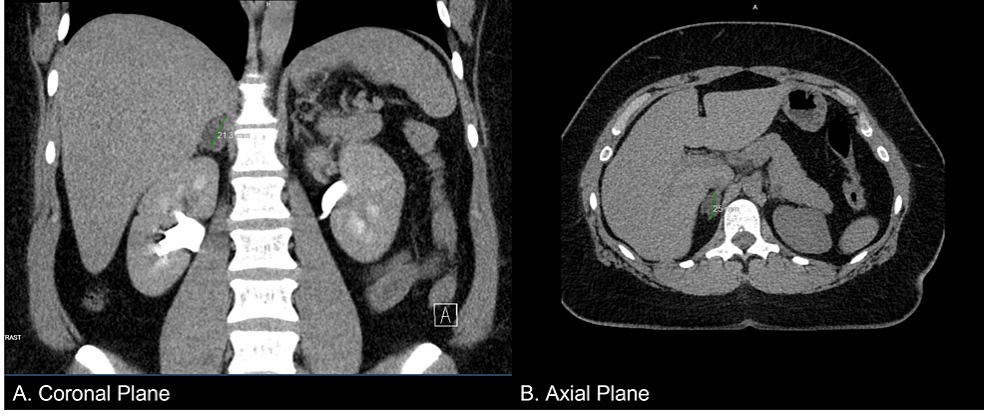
Abdominal CT scan showing a nodule in the right adrenal gland measuring 2.1 x 1.5 x 2.5 cm
